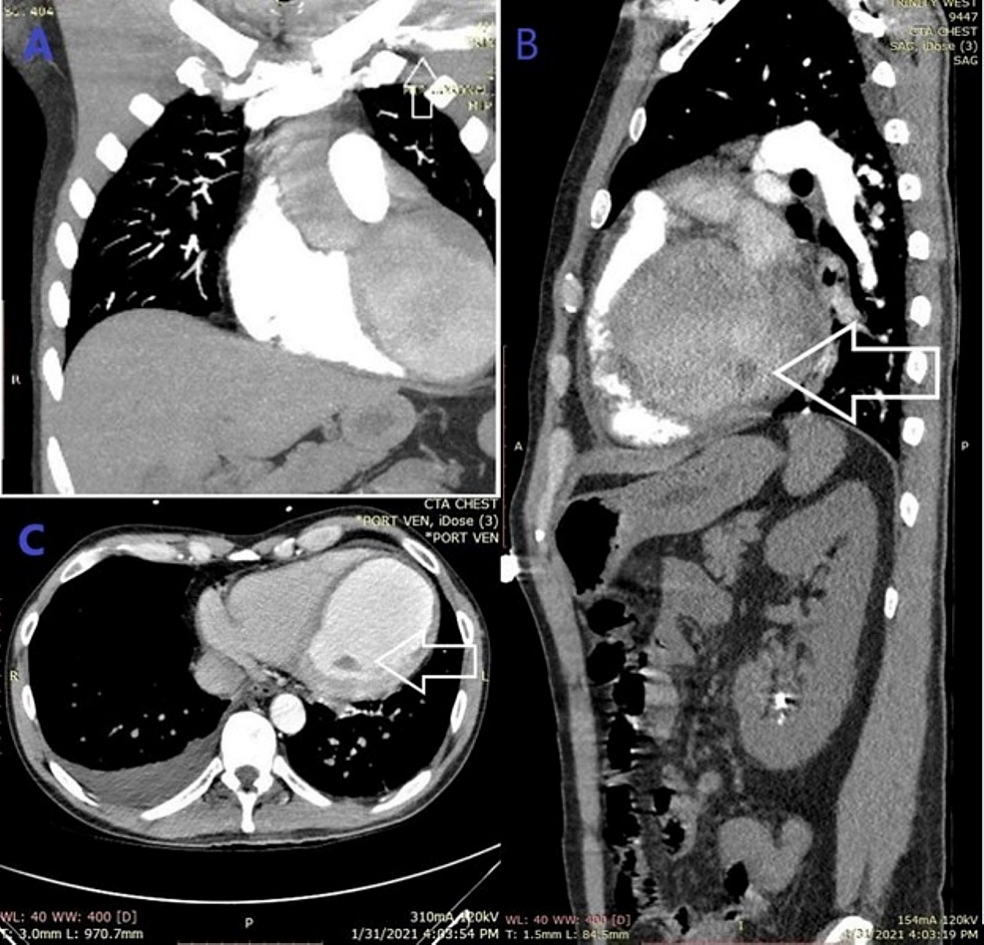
CTA of the chest. (A) Coronal view with partially occluding left subclavian vein thrombus. (B) Sagittal view with intraventricular filling defect. (C) Axial view with intraventricular filling defect CTA: computed tomography angiography
